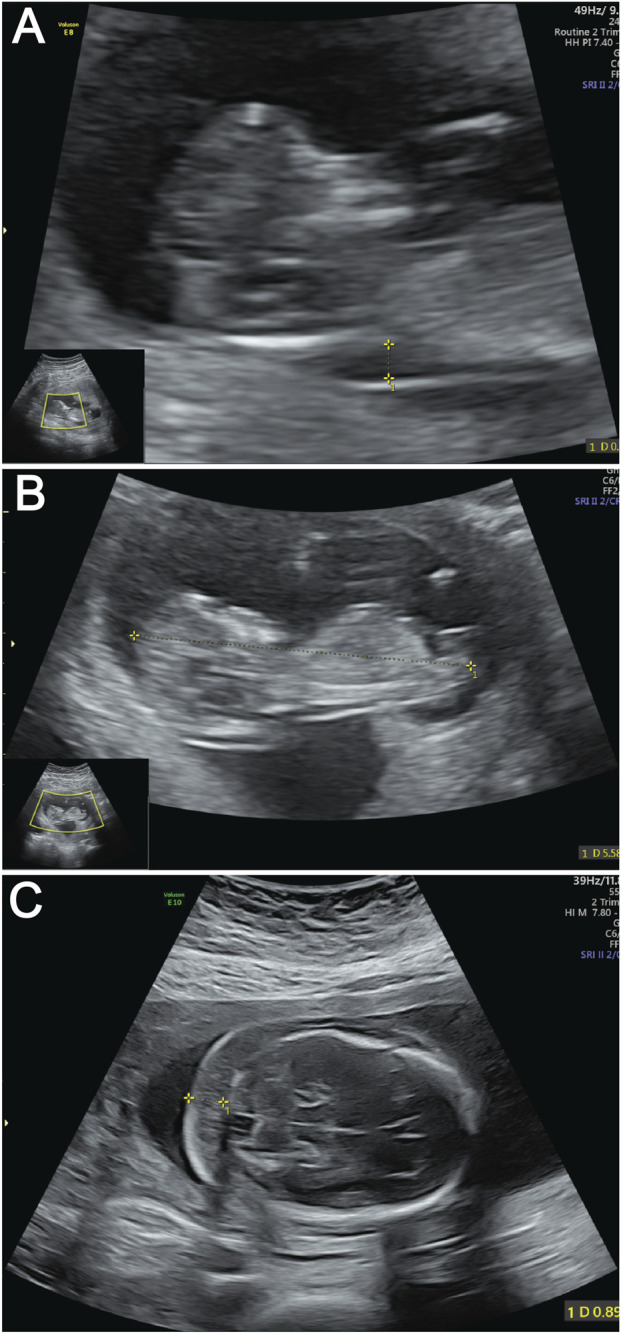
Ultrasound images of fetus demonstrating key anatomical views. (A) Ultrasound measurement of NT thickness. Yellow calipers mark the NT measurement points; (B) Fetal CRL measurement by ultrasound. The dotted line between yellow calipers indicates the CRL. (C) Ultrasound evaluation of NF thickness. Yellow calipers demarcate the NF measurement area.
